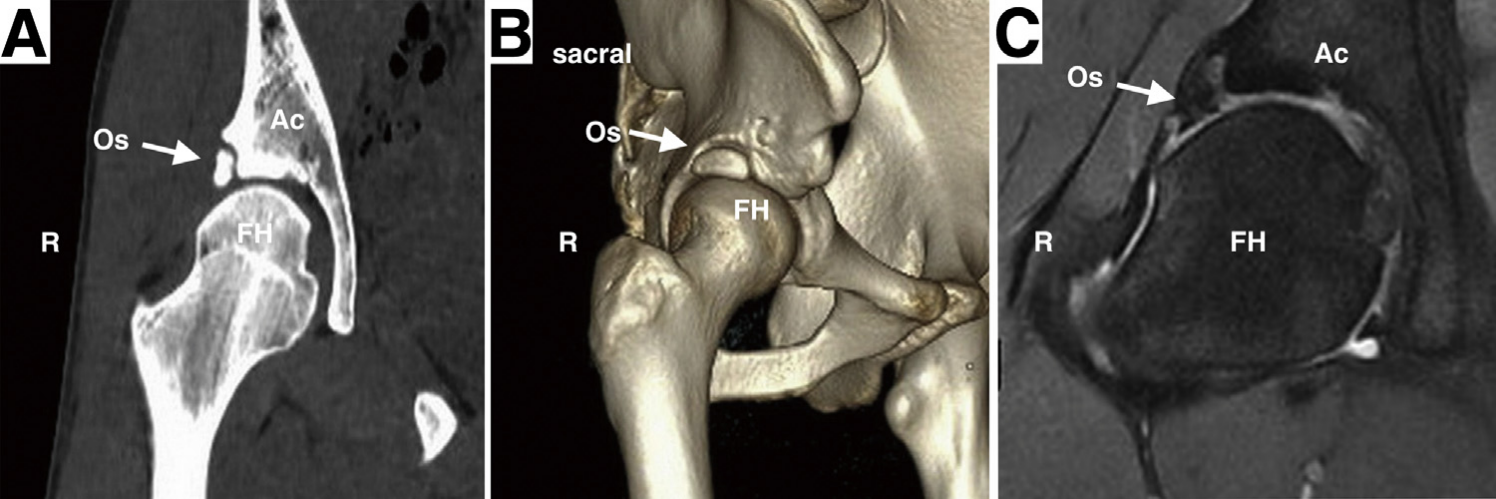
Preoperative CT and MRI of the right hip displaying os acetabuli (white arrow) and related injury. (A) Two-dimensional reconstructed coronal CT image showing the a bone fragment isolated from the acetabular rim. (B) Three-dimensional reconstructed CT image showing the spatial location of the Os acetabuli. (C) MRI image showing os acetabuli and related chondrolabral injure. (Ac, acetabulum; CT, computed tomography; FH, femoral head; MRI, magnetic resonance imaging; Os, os acetabuli.)
